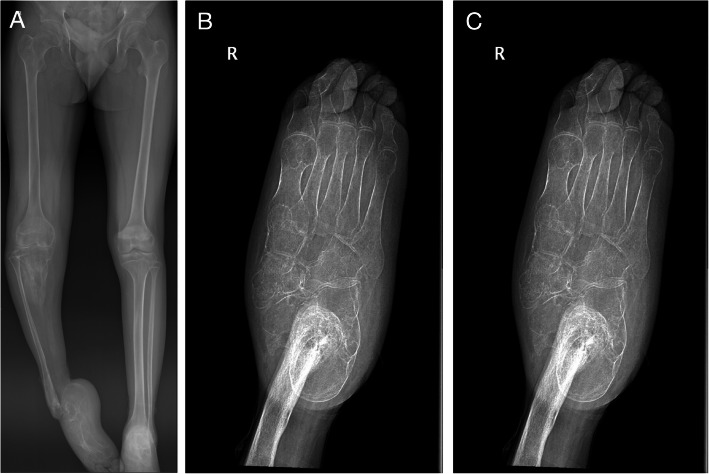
a, X-ray AP view bilateral lower limbs. b, c, X-ray of foot
